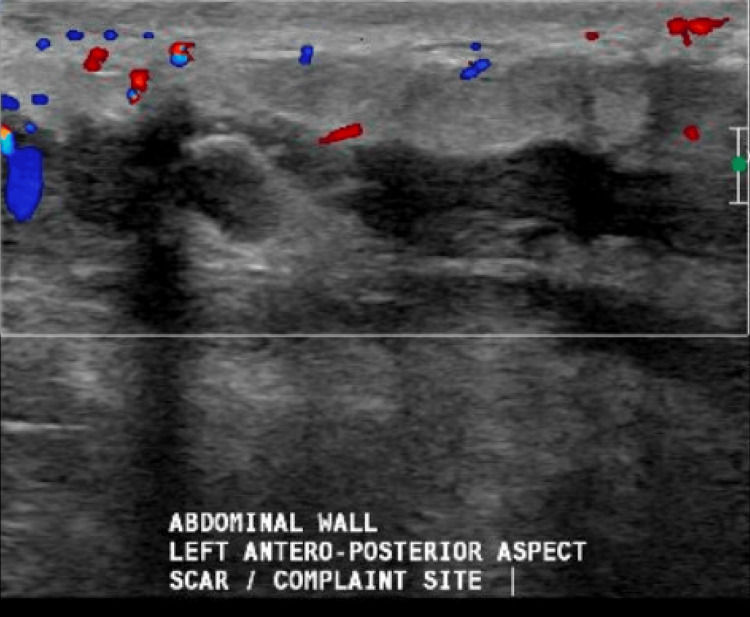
Ultrasound of the abdominal wall during the first visit to ED reflecting pus accumulation
